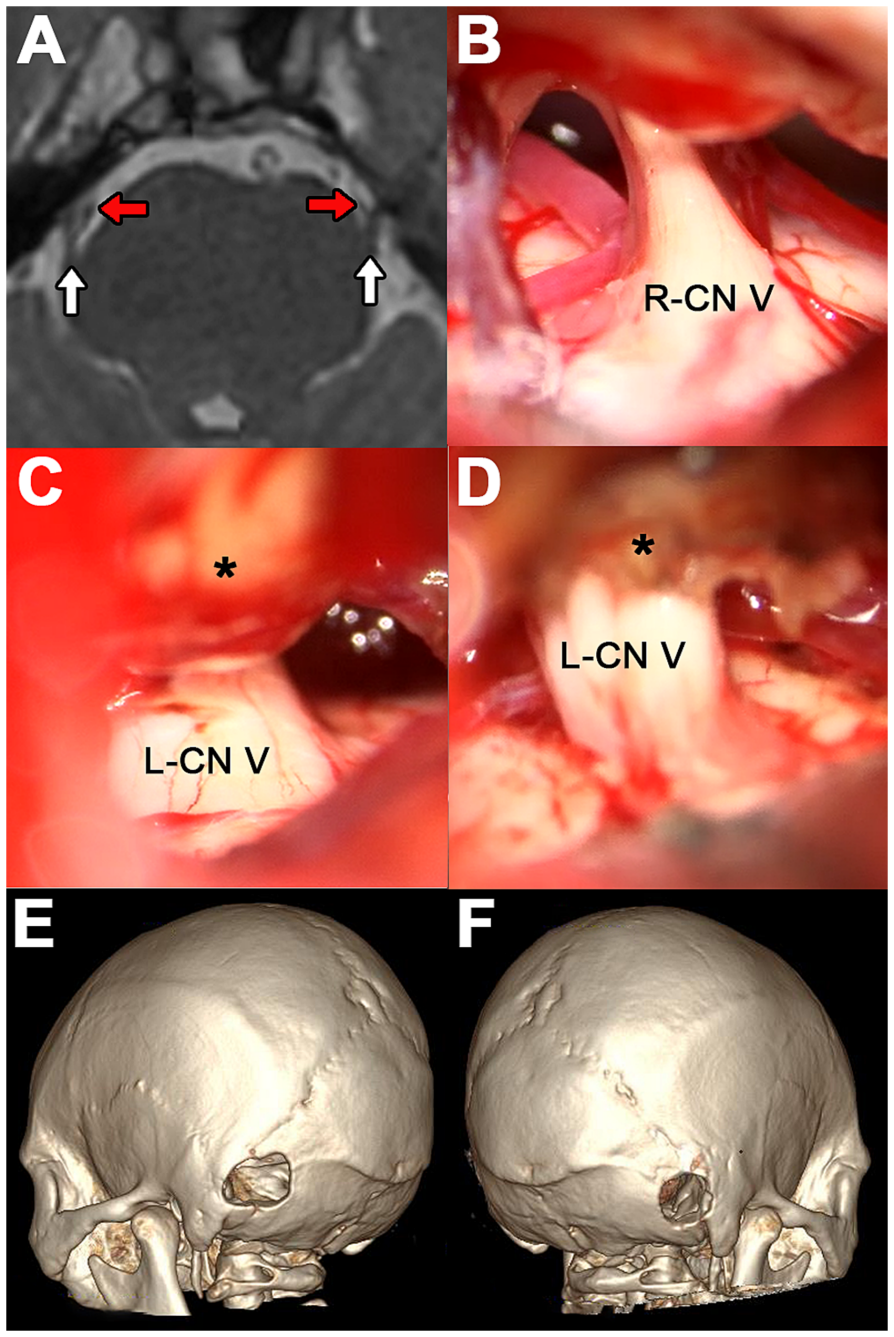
Case 1. (A) Axial 3D-FIESTA MRI at the level of the pons. The white arrows indicate the cisternal portion of CN V and the red arrows the contact vessels. (B) Surgical exploration on the right side showing SCA as the culprit vessel. (C) Suprameatal tubercle (asterisk) obstructing visualization of the left CN V. (D) Left-sided surgical exploration showing drilled suprameatal tubercle (asterisk) and redundant SCA as the offending vessel. (E,F) 3D reconstruction CT scan showing left and right retrosigmoid craniotomy, respectively.
